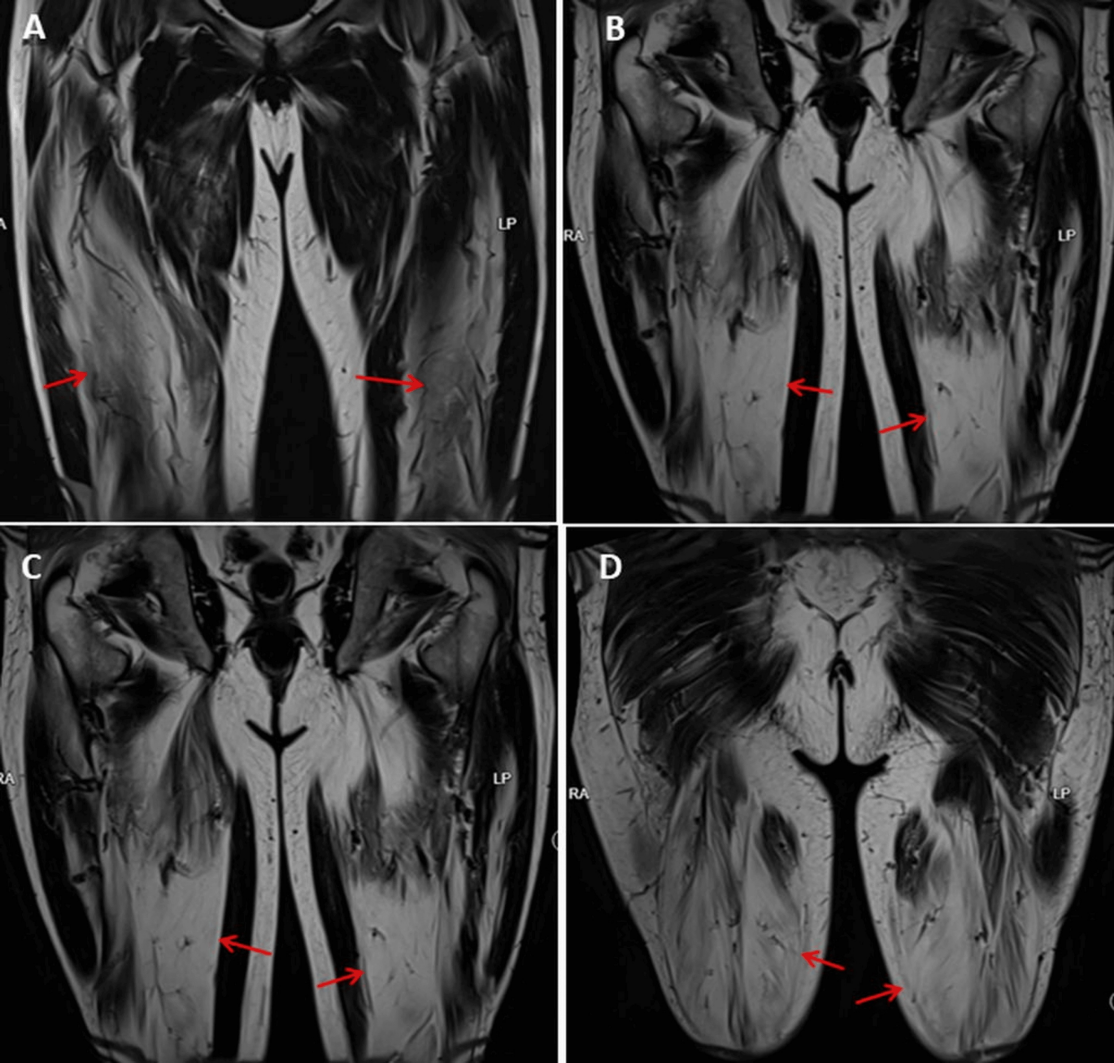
Muscle MRI (T2-weighted imaging, coronary view, Dixon technique, fat suppression) showing hyperintensity (fatty infiltration) in the vastus intermedius (A, arrows), rectus femoris (B, arrows), vastus lateralis (C, arrows), and hamstring muscles (D, arrows).
